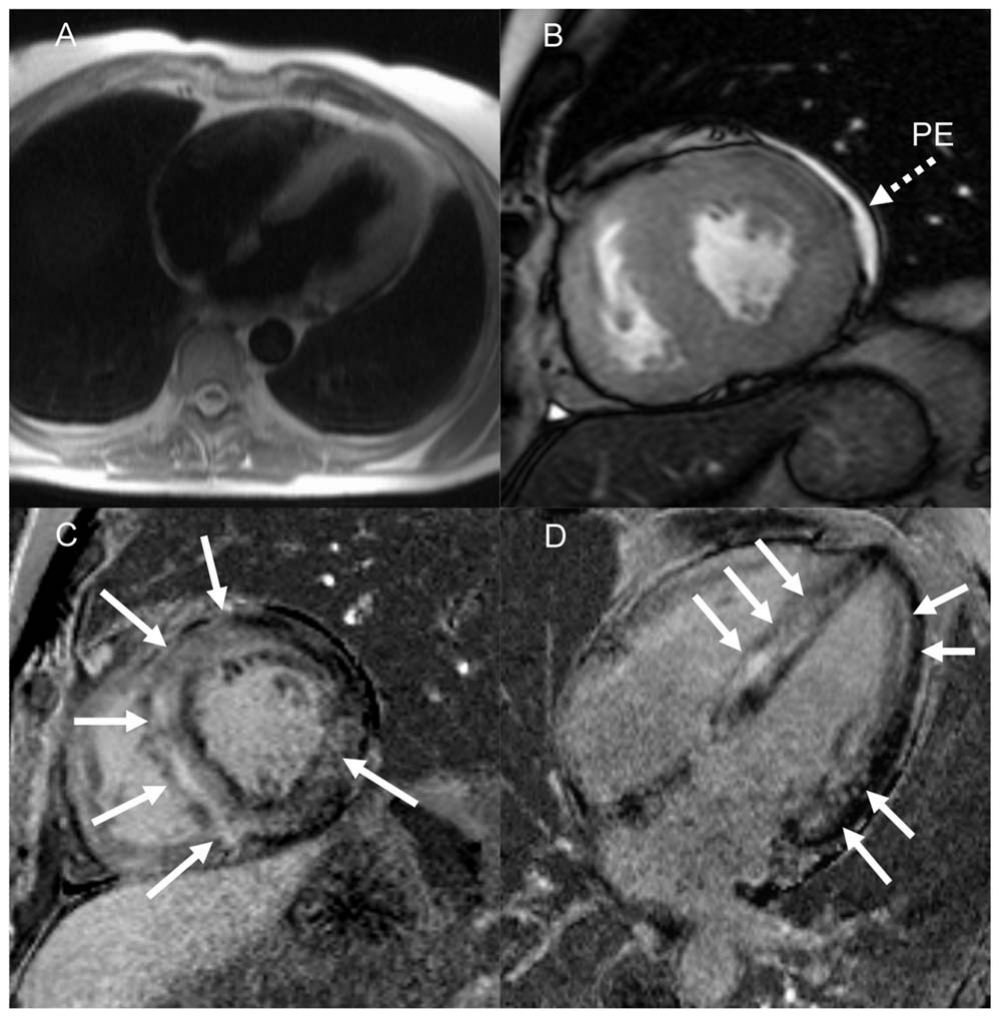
Cardiac magnetic resonance imaging in a patient with AL amyloidosis and LV hypertrophy. A (transverse T2 haste image) and B (short axis cine image) demonstrate left ventricular hypertrophy and minor pericardial effusion (dash arrow). C and D show late gadolinium enhancement images (short axis and horizontal long axis) presenting diffuse LE (solid arrows) in the left ventricular walls. LE: late enhancement; PE: pericardial effusion.
